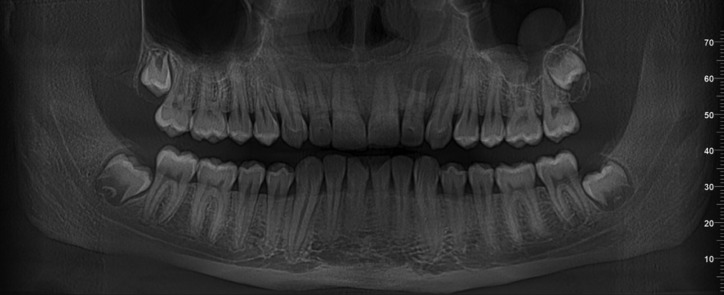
Preoperative panoramic view X-ray reconstruction from a CBCT. It is possible to observe the size of two cystic lesions involving teeth 12 and 22. Also, four impacted third molars present and an incidental left maxillary sinus mucosal cyst finding.
